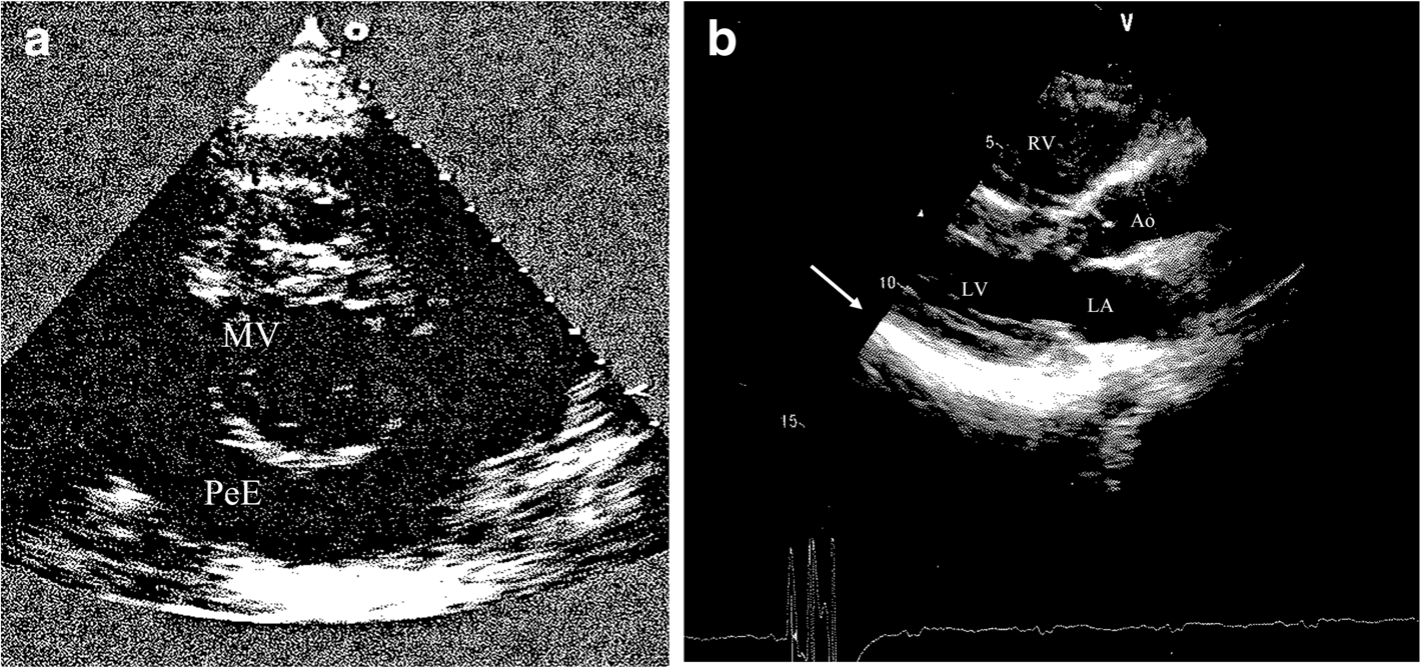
a Parasternal short-axis view showing large pericardial effusion (30 mm): > 20 mm “eco-free space” on echocardiogram is indicative of large pericardial effusion. MV: mitral valve; PeE: pericardial effusion. b Mild pericardial detachment on right atrium (white arrow) without sign of compression on 1-month echocardiographic follow-up (parasternal long-axis view). Ao: aorta; LA: left atrium; LV: left ventricle; RV: right ventricle
